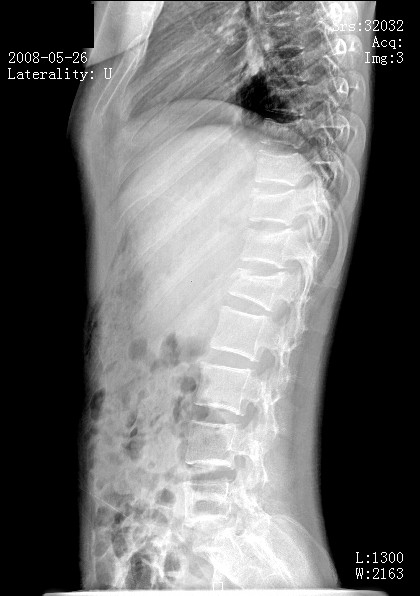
Pre-operative radiograph (lateral view) with thoracolumbar vertebral collapse.
